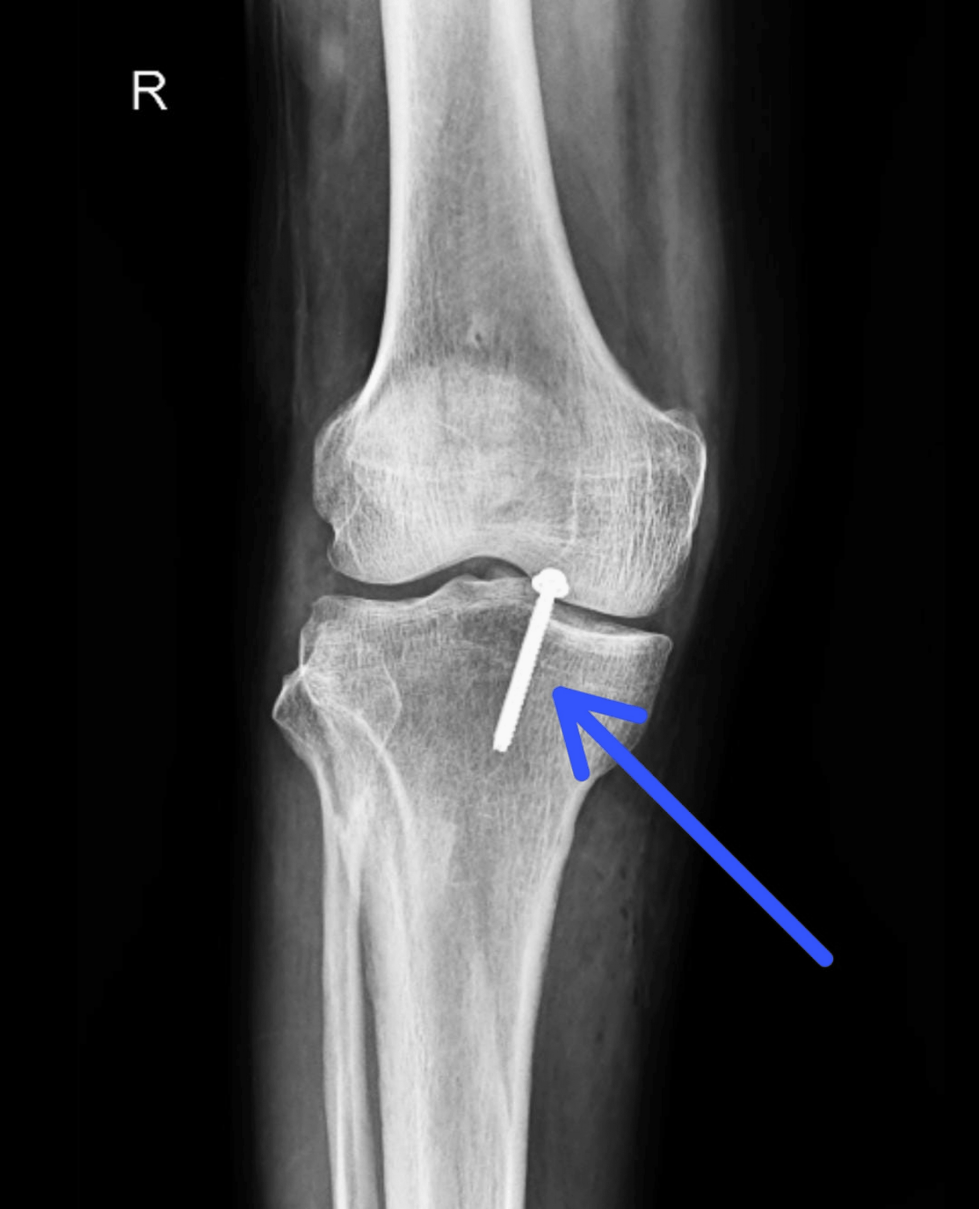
Anteroposterior (AP) view of the X-ray shows a right knee with a screw used to fix an avulsion of the posterior cruciate ligament (PCL).
